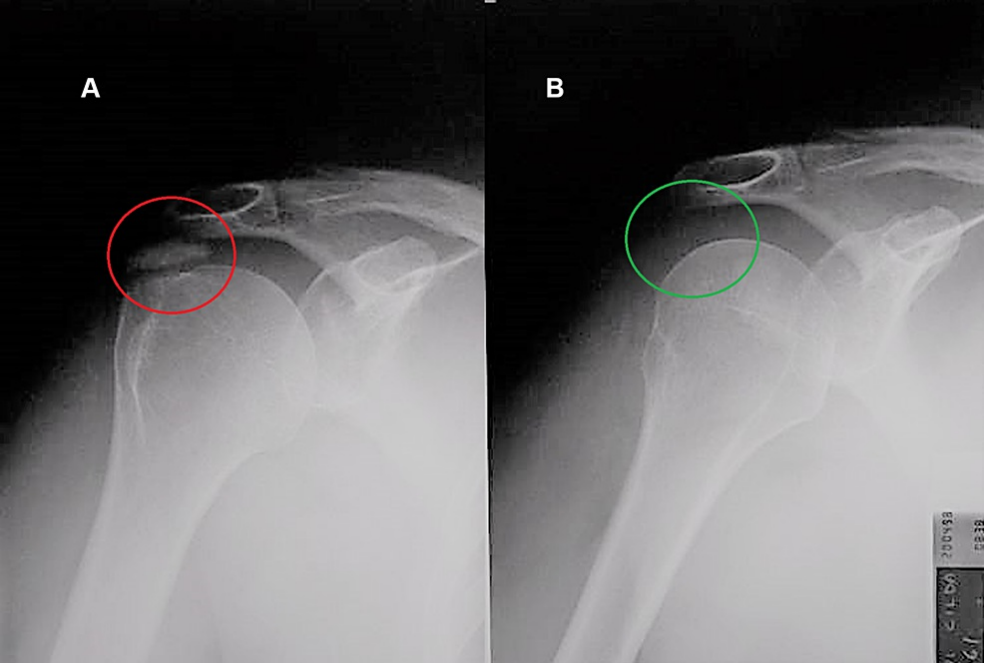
X-rays before and after REAC treatments. The calcifications are highlighted in red (A), and the disappearance of the calcification is highlighted in green (B). REAC: radioelectric asymmetric conveyer
